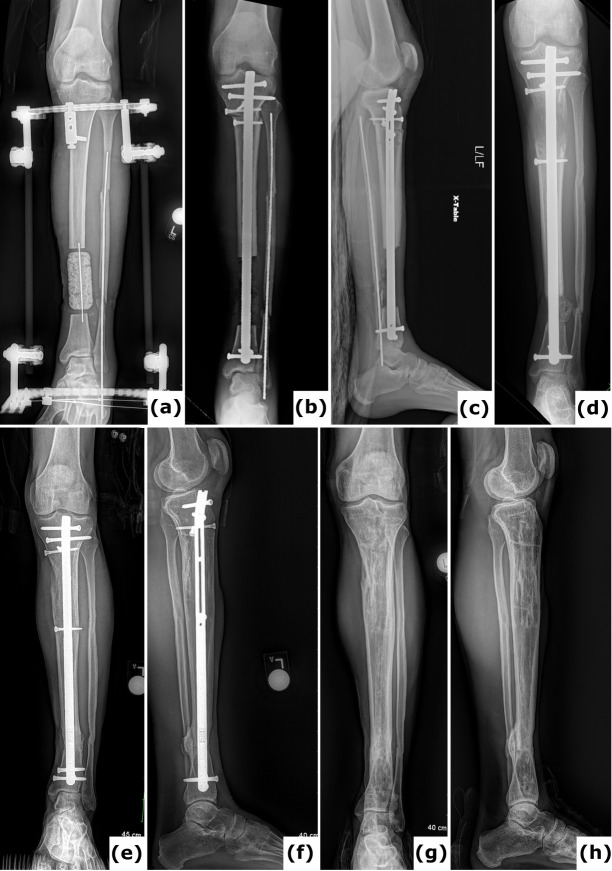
Bone transport nail. (a) Anteroposterior radiograph of a 26-year-old patient with a Gustilo-Anderson type III open tibia and fibula fracture, including 95 mm bone loss. In the initial surgery, a temporary PMMA spacer was placed at the defect site and the bone ends were prepared for bone transport. Provisional fixation was achieved using initial hybrid spanning fixation and an intramedullary wire in the fibula. (b–c) Anteroposterior and lateral radiographs display application of a bone transport nail with proximal and distal locking pegs, and required poller screw fixation to minimize drift during transport. (d) Anteroposterior radiograph demonstrates regenerate formation after a 2-week latency period and distraction at 0.6 mm d^−1^. (e–f) Anteroposterior and lateral radiographs document progressive healing subsequent to open docking. (g–h) Anteroposterior and lateral radiographs indicate solid and mature healing following removal of the bone transport nail 2 years post-injury.
